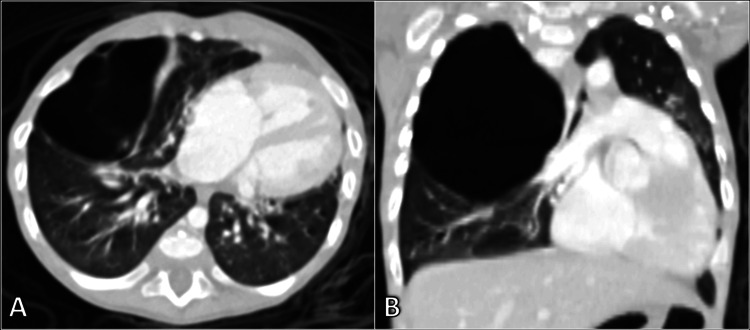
Contrast-enhanced CT images of the chest demonstrating extensive congenital pulmonary airway malformation. (A) Axial CT image showing a large multicystic lesion occupying almost the entire right upper and middle lobes, with multiple variable-sized cystic spaces replacing the normal pulmonary parenchyma. No solid soft tissue component or abnormal vascular structures are identified. The lesion produces significant mass effect with compression of adjacent lung tissue and contralateral mediastinal shift.
